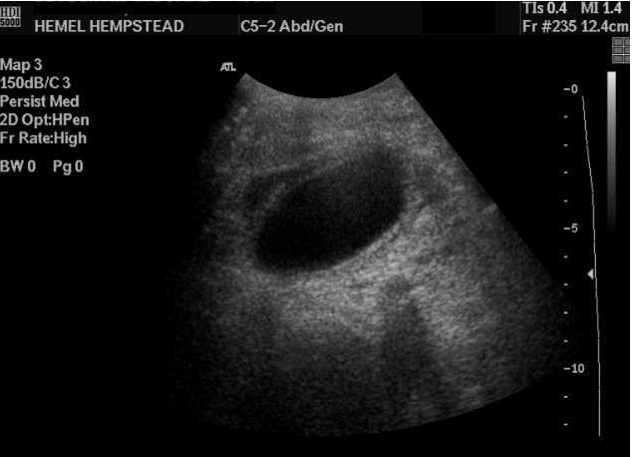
Abdominal ultrasound. A distended, thick-walled gallbladder with no gallstones and a cuff of pericholecystic fluid were revealed.
